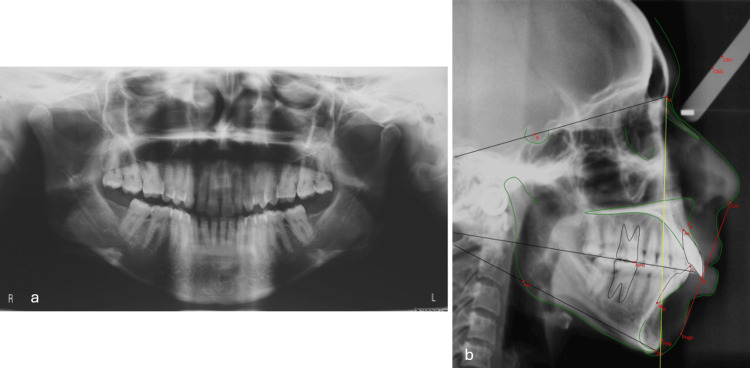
Pre-treatment radiographic records. (a) Panoramic radiograph. (b) Lateral cephalometric radiograph.
